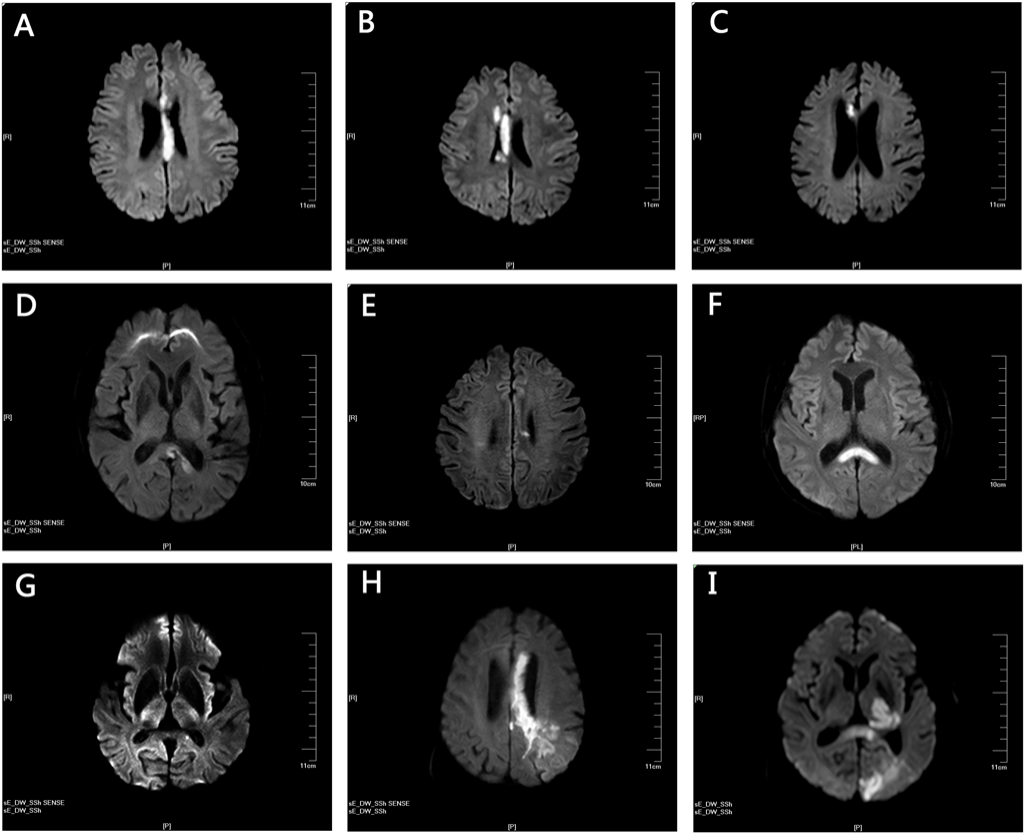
Representative images of pure and complex corpus callosum (CC) infarction. (A) to (G) shows all individual cases presenting with ischemic lesions restricted to the CC. (A) and (B) DWI showing infarctions in CC genu, body and splenium. (C) infarctions situated in the right body and genu of CC. (D) ischemic lesions were detected in the left splenium. (E) small infarction in the left CC body. (F) bilateral splenium infarctions. (G) very small infarction of the left splenium. (H) and (I) provide representative examples of patients showing additional ischemic lesions outside the CC. (H) infarctions involving the left CC genu and body as well as the occipital-parietal areas. (I) lesions located in the occipital lobe, the left thalamus as well as the left CC genu and body.
